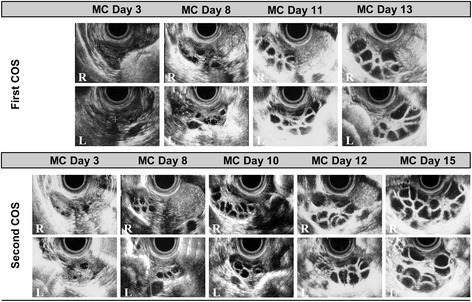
Follicular development following controlled ovarian stimulation (COS). Ovarian response was monitored through the use of transvaginal ultrasonography during COS. During the first COS, measurable follicles were not observed in either ovary on day 3 of the menstrual cycle (MC). On the same day, COS was initiated with daily administration of 225 international unit (IU) recombinant follicle stimulating hormone (rFSH). Follicular development and increased progesterone (P_4_) concentrations were observed, but estradiol (E_2_) concentrations were low on day 11. Although the right (R) and left (L) ovaries had over 30 follicles of 15–19 mm on day 13, serum E_2_ concentrations were low at 484 pg/ml and P_4_ concentrations were high at 2.09 ng/ml. COS was canceled due to the hormone concentration which did not reflect those of numerous follicular developments. From the day when the first COS was canceled, the patient took 10 mg synthetic progesterone (SP) orally for the next 14 days. The patient experienced withdrawal bleeding, and several small follicles were observed in both ovaries on day 3. From the same day, the daily dosage of 175 IU rFSH was administered for the first 5 days, followed by 200 IU for the remaining treatment period. Similar to the response seen at the first COS, high serum P_4_ concentrations, 2.11 ng/ml, were observed; however, serum E_2_ concentrations, 701 pg/ml, did not reflect those of numerous follicular developments
